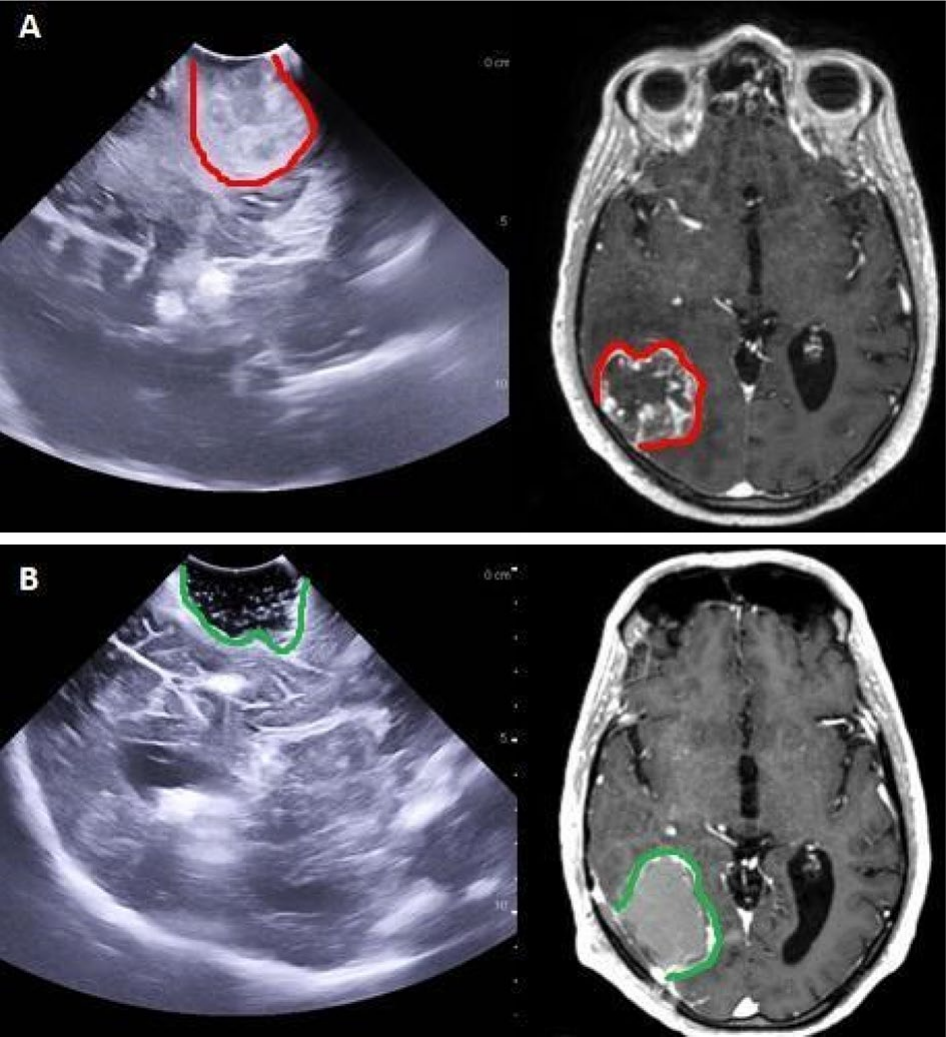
This figure shows a case of a right temporal GB operated with the perilesional dissection technique. In this picture it is possible to understand how US and MRI can look like similar pictures
